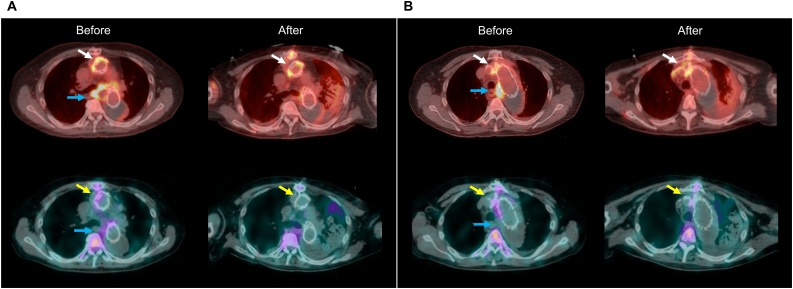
Comparison between FDG-PET/CT and Ga scintigraphy after esophagectomy. Blue arrows show abnormal uptake of each tracer around aorto-esophageal fistula. White arrows show abnormal uptake of FDG in the infected lesions whose cultures were positive for methicillin-resistant Staphylococcus epidermidis, and yellow arrows abnormal uptake of Ga in these same lesions (A; distal anastomosis of the ascending graft, B; aortic arch). After esophagectomy, there were no significant Ga uptake at those infected lesions, while high FDG uptake remained at those infected lesions (white and yellow arrows).
